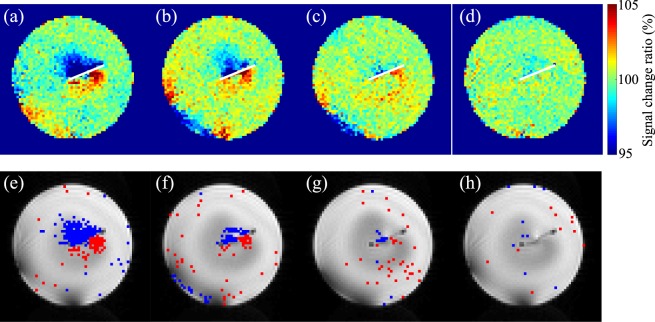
MR images of tiny magnetic field measurements. *ω*/2*π* and *ω*_sl_/2*π* were 96 Hz, and *α* and *ϕ* were set to 110° and 0°, respectively. Each image was averaged with 10 images. The current-dipole moment was (a) 500 nAm, (b) 250 nAm, (c) 100 nAm, and (d) 50 nAm. The white line indicated the dipole electrode. Two-sided *t*-test results of (a–d) were shown in (e–h), The threshold was *p* < 0.01. The negative and positive signal changes were indicated with blue and red pixels, respectively.
